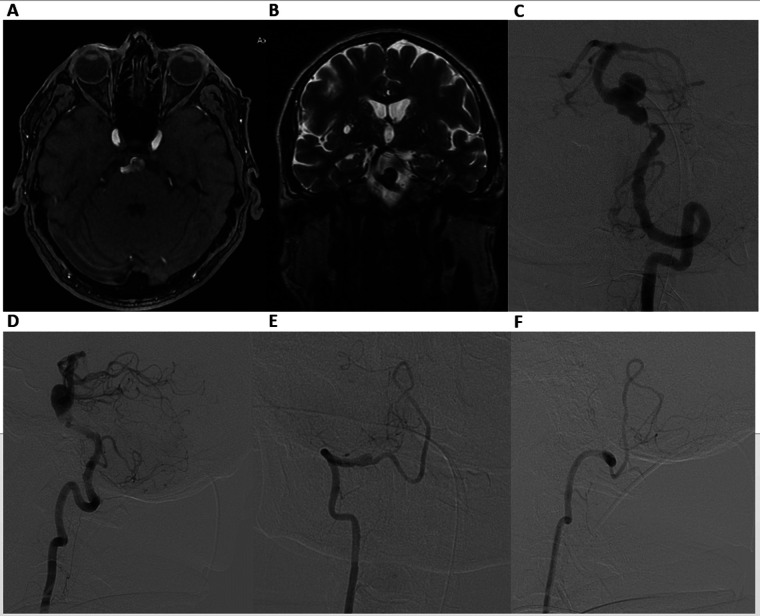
Axial time-of-flight (TOF) MR angiography (A), coronal T2-weighted MRI (B), demonstrating a basilar artery aneurysm. Digital Subtraction Angiography. Frontal (C) and lateral (D) views of the left vertebral artery, showing a wide-neck aneurysm in the inferior third of the basilar artery, accompanied by nearly 90% preaneurysmal stenosis. Frontal (E) and lateral (F) views of the right vertebral artery, revealing V4 segment occlusion distal to the right posterior inferior cerebellar artery.
